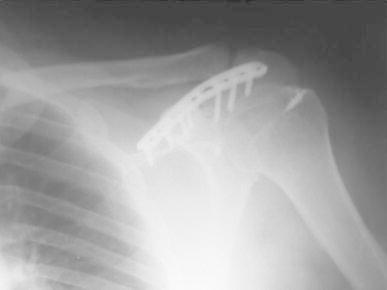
Follow-up roentgenogram, postoperative 6 months
